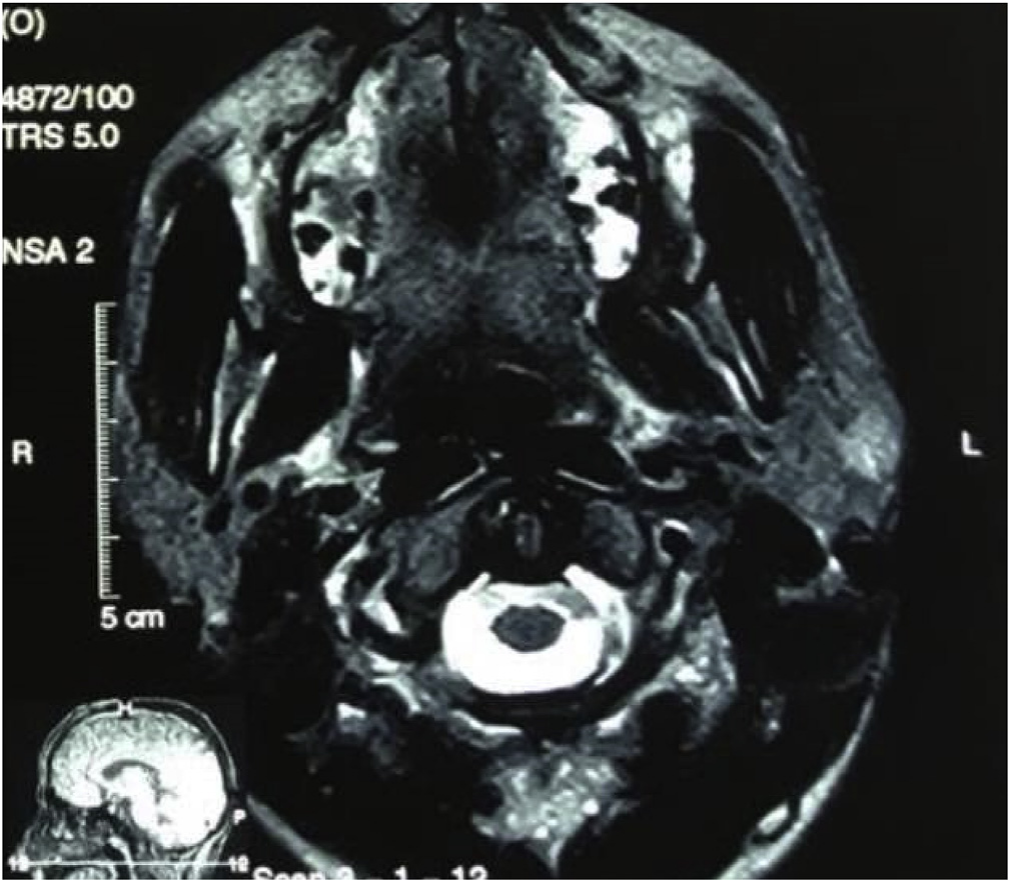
MRI T1 transverse section: visualisation of the pseudoaneurysm mimicking an intra-parotid mass.
